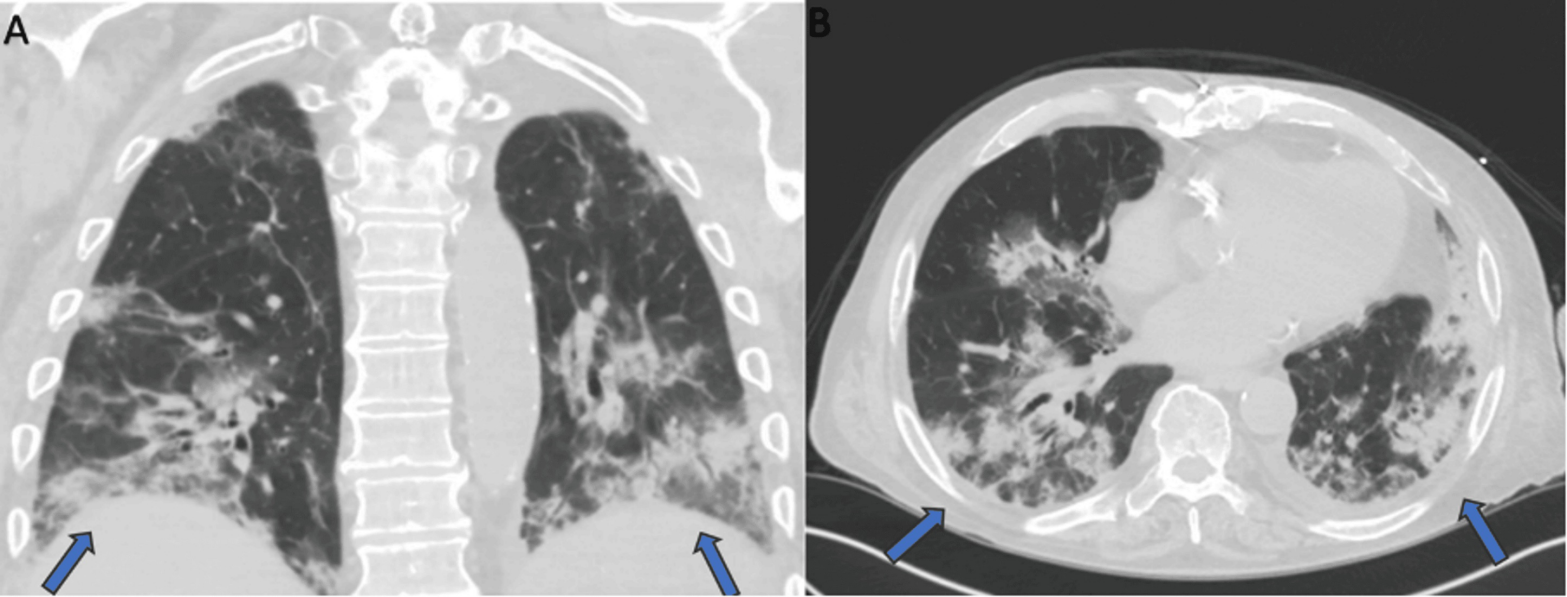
Chest CT showing patchy bilateral alveolar and ground-glass opacities (arrows), which may represent acute eosinophilic pneumonia. A: coronal view; B: axial view
